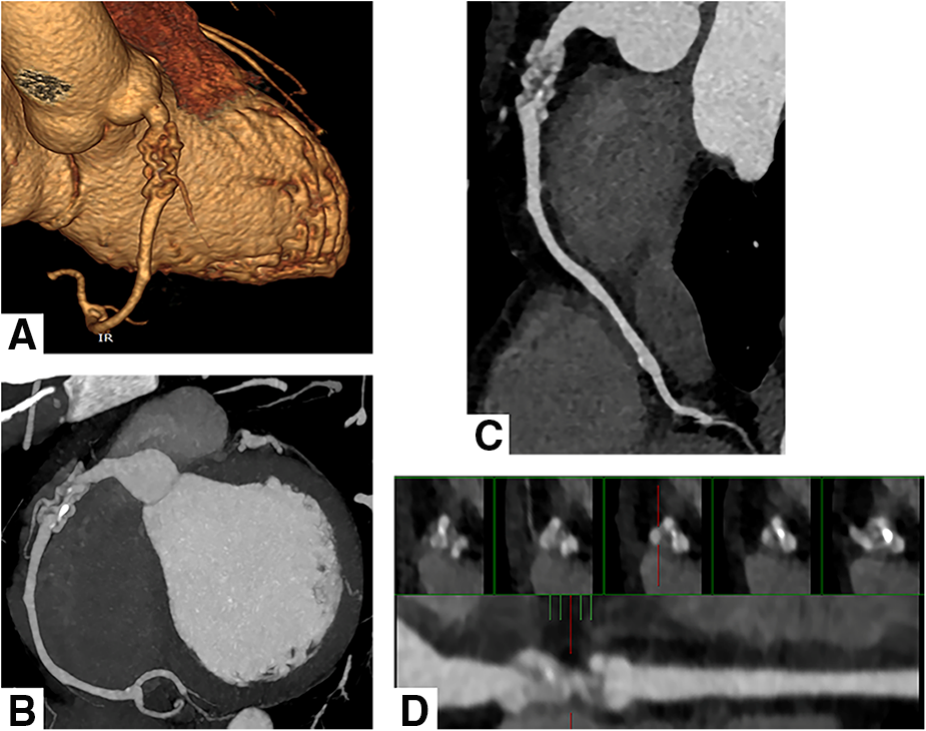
Computed tomography coronary angiography shows the WCA in the right coronary artery. Volume rendering reconstruction (A), Maximum intensity projection (B) and Curved multiplanar reconstruction (C) revealed multiple twisting channels in the proximal segment of the right coronary artery and merged again into a normal conduit. Small patchy calcification was observed in the structure. (D) Consecutive cross-sectional images at the lesion site showed twisting and multiple small channels.
